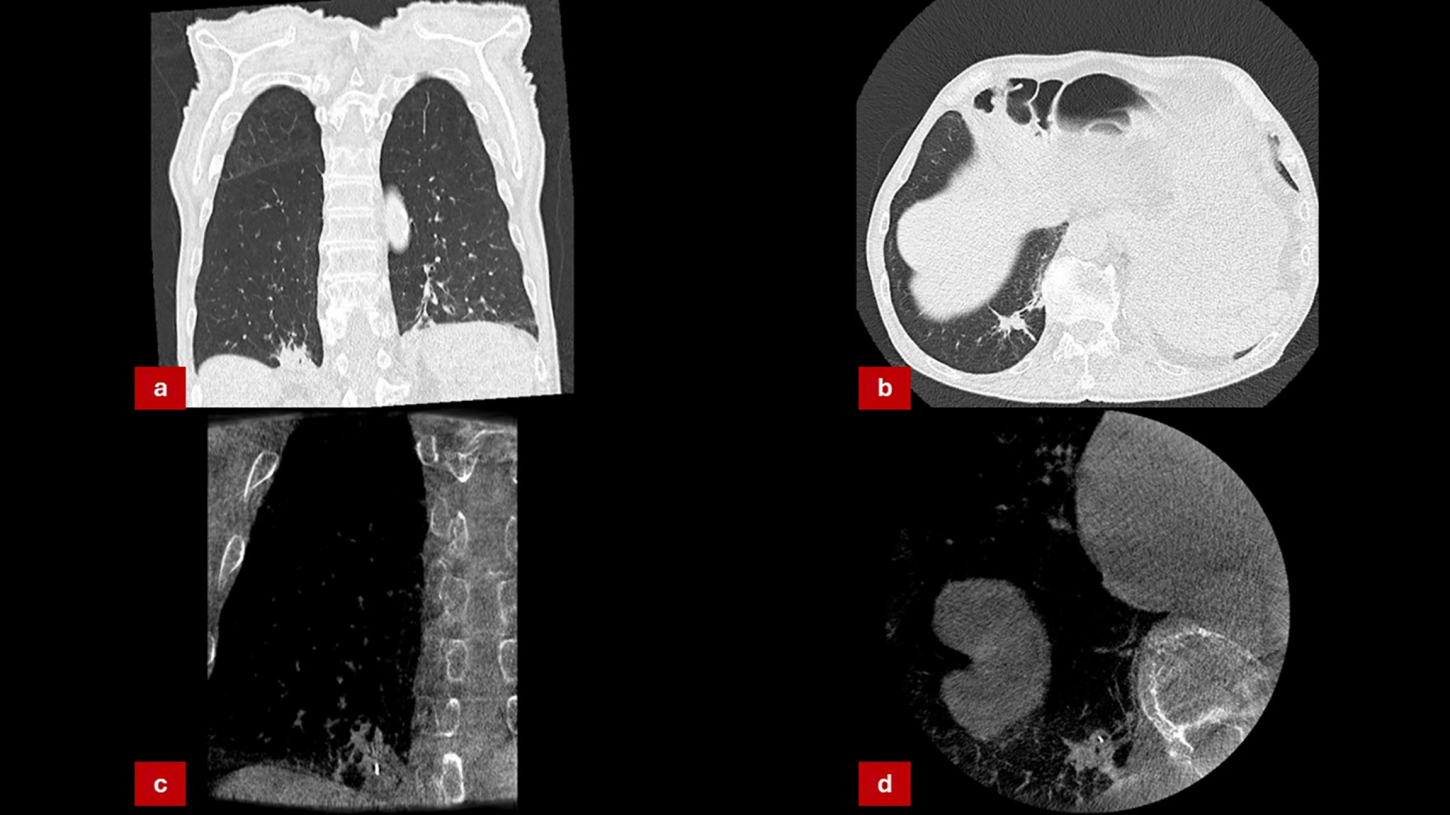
(a, b) CT scans revealing a solitary pulmonary lesion within the left lower lobe. (c, d) CBCT‐Imaging done during bronchoscopy confirming appropriate tool placement.
